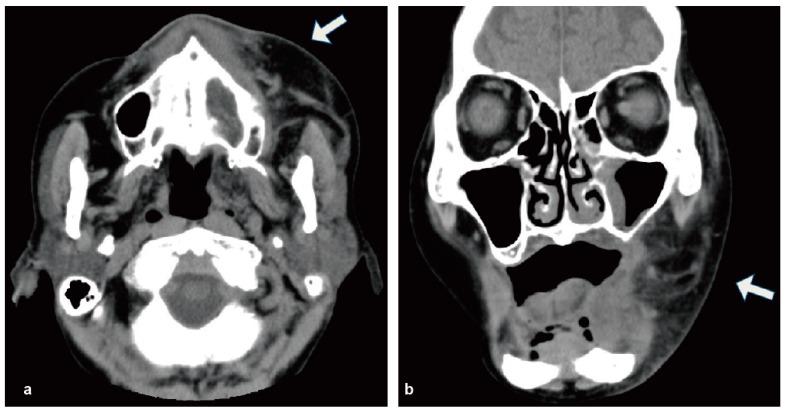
Enhanced CT revealed the soft tissue swelling widely extended to the left submandibular space without abscess formation (arrowhead). (a) Axial scan; (b) coronal scan.
